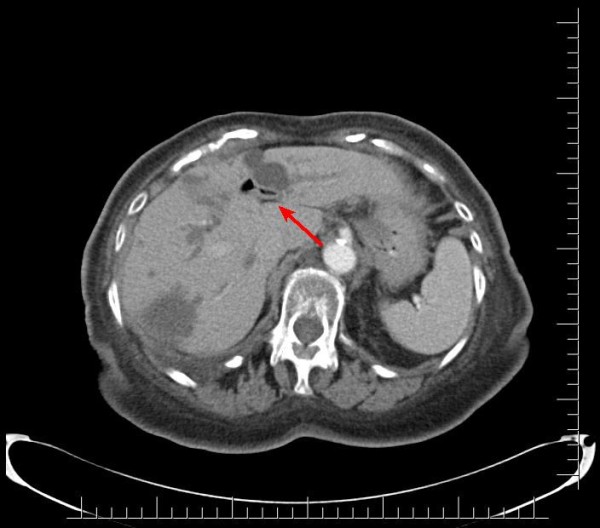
Axial computed tomography showing a hepatic cyst infringing on the ductal system.
